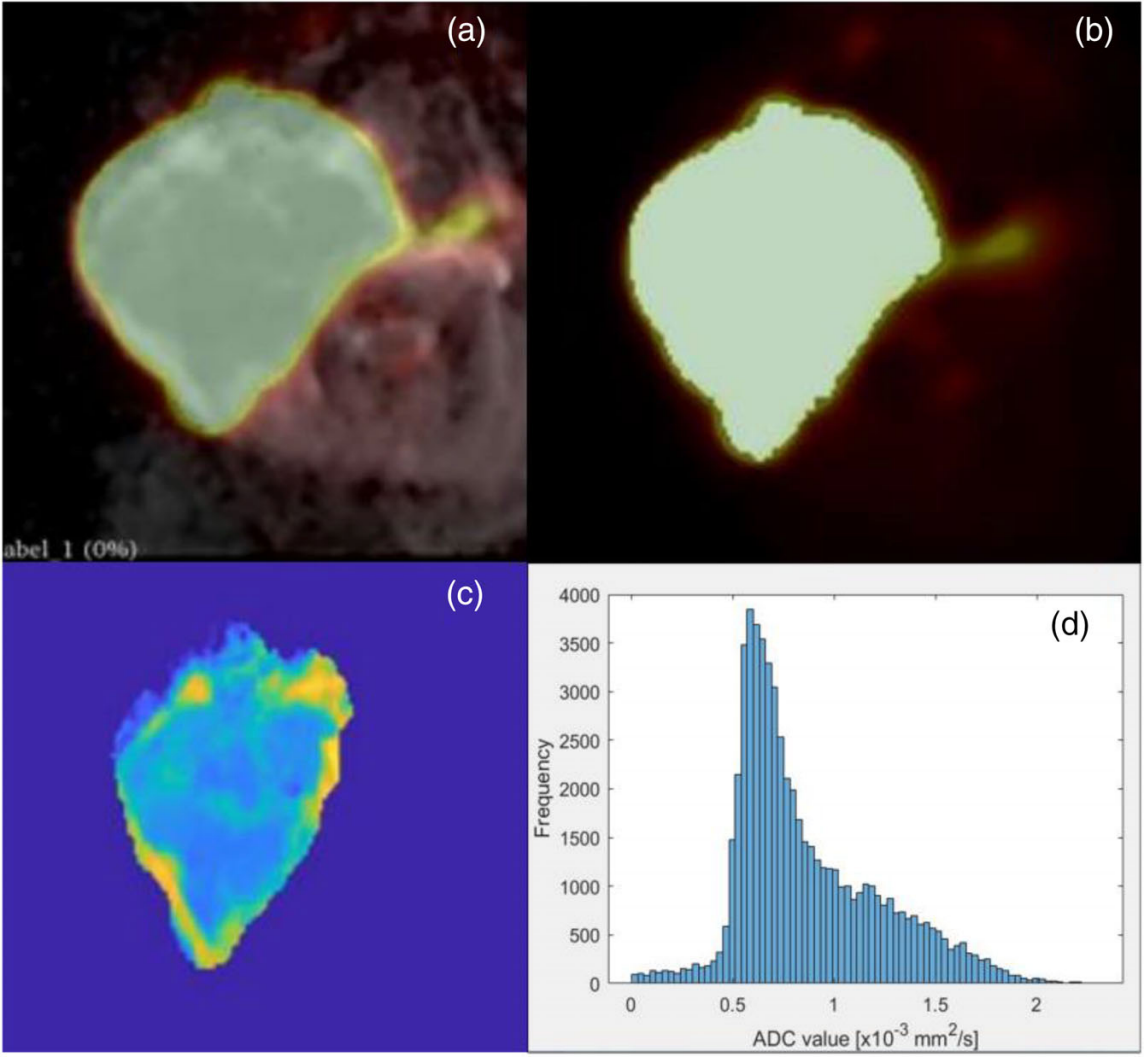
ADC image overlaid by [^18^F]‐FDG PET AC image and tumor contorting (a) and its corresponding binary mask volume (b). Segmented tumor mass (c) and its corresponding ADC histogram (d).
